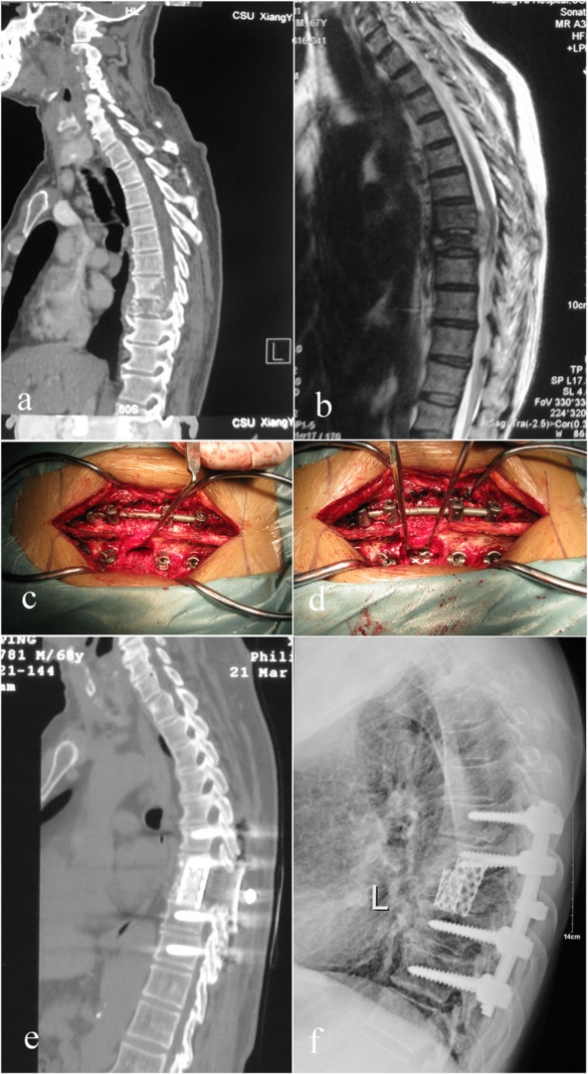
Case number 25. Preoperative image evaluation. a Computed tomography (CT) and b magnetic resonance imagery (MRI) showing T8–T9 involvement. c, d Operation technique and specially formed titanium mesh cages. e, f At final follow-up, the internal fixation was in good shape and interbody fusion had been obtained, without signs of tuberculosis recurrence
